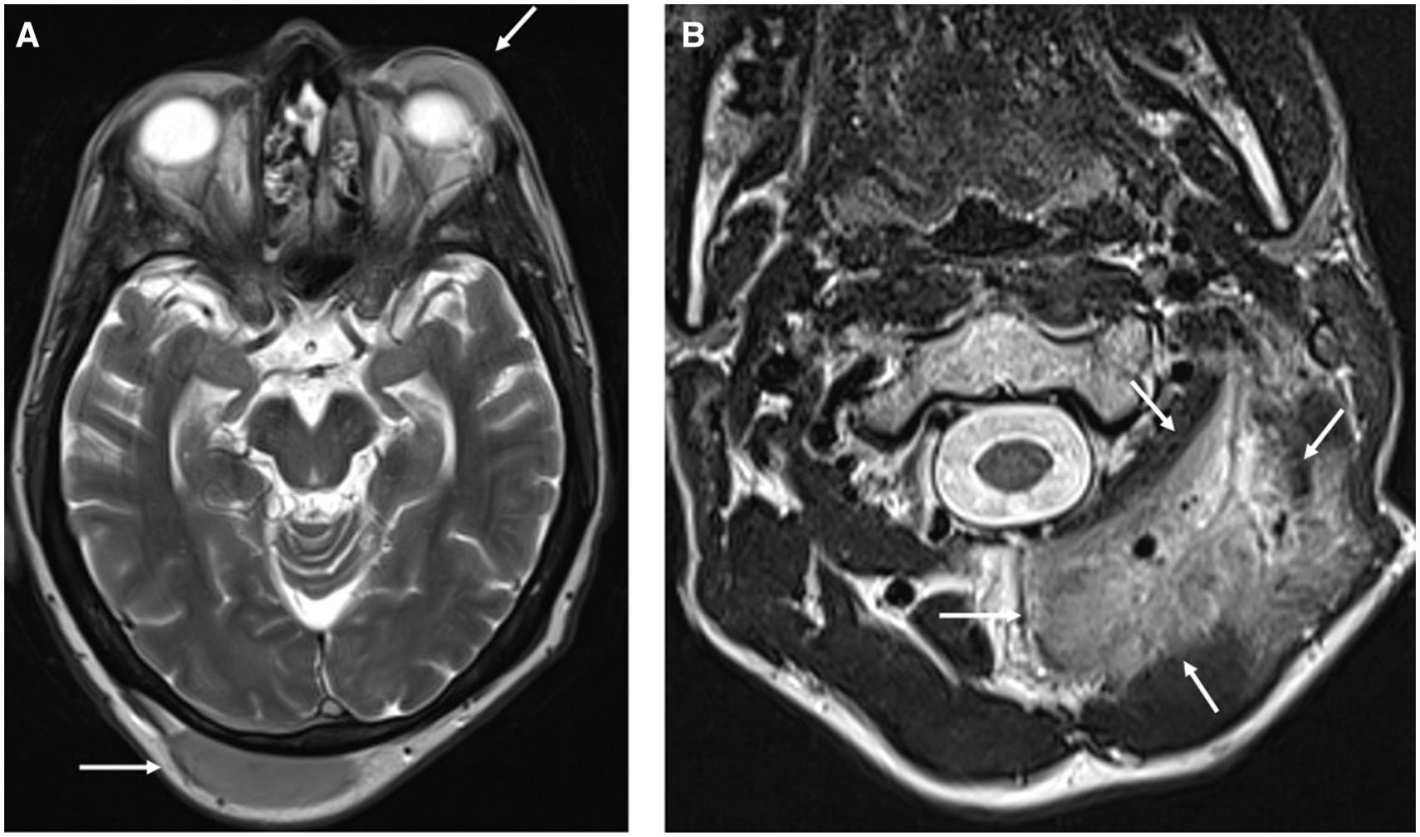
EMPs of the head and facial soft tissues. (A) Axial T2-weighted MRI of the brain show EMPs (white arrows) arising from the right occipital scalp and left upper eyelid (no globe/post-septal involvement). EMP in the perivertebral compartment. (B) Axial T2-weighted MRI shows an intermediate signal lesion infiltrating trapezius and the paraspinal muscles of the left upper neck (arrowed).
